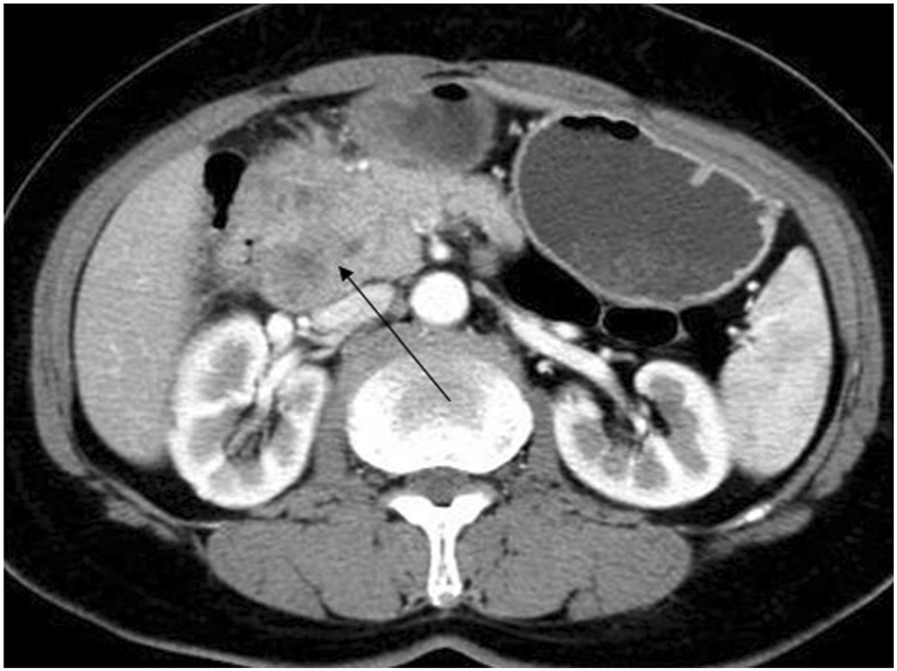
Abdominal computed tomography (CT) showed an 8 cm × 10 cm lobulated solitary mass encircling the duodenum with low attenuation and inhomogeneous enhancement (arrow).
